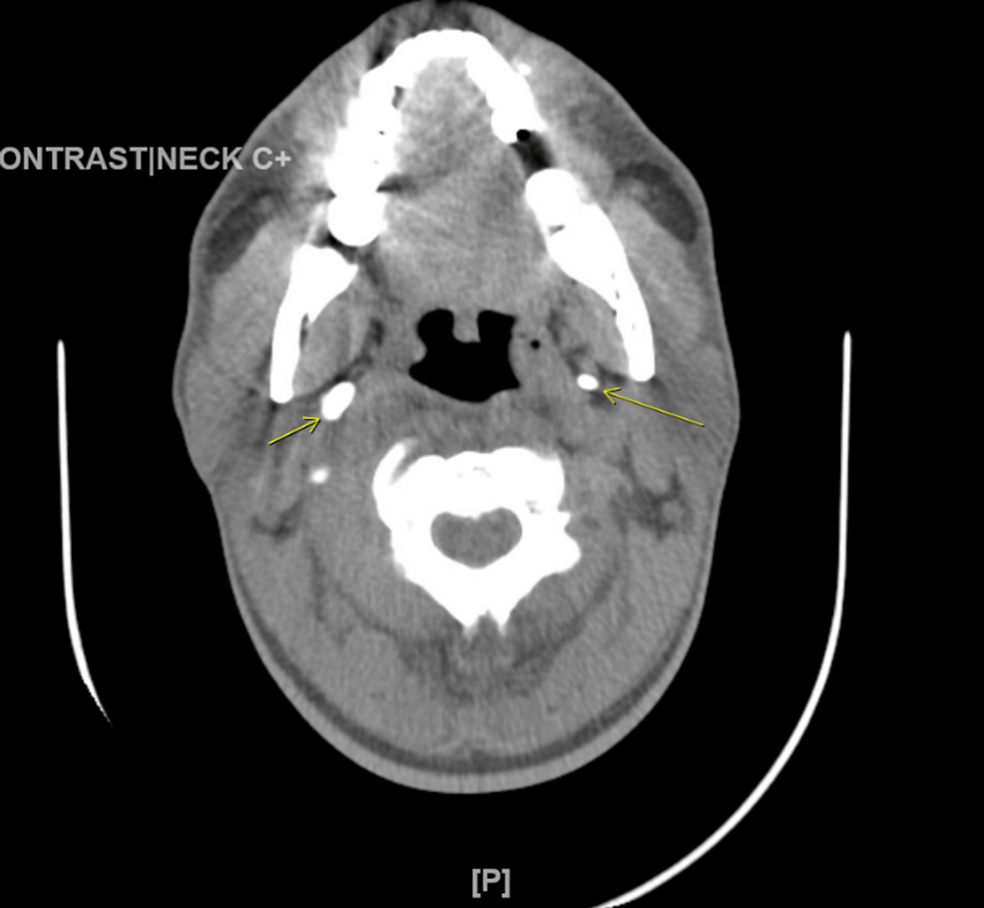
CT neck without contrast before decannulation Transverse view at the C3/C4 vertebra level showing ossification of the bilateral stylohyoid ligaments
